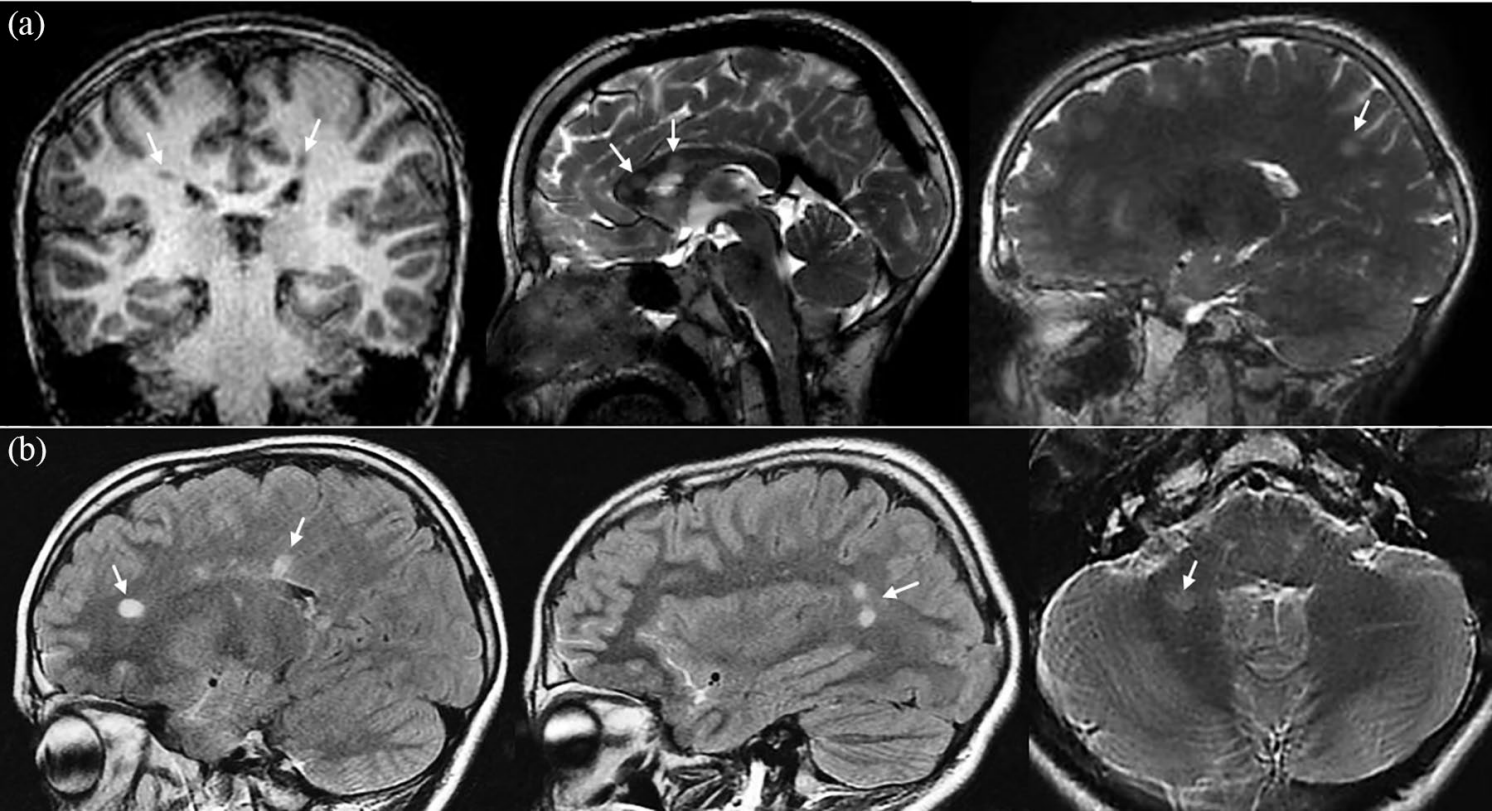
MR images at baseline and follow-up. (a) One coronal T_1_-weighted
